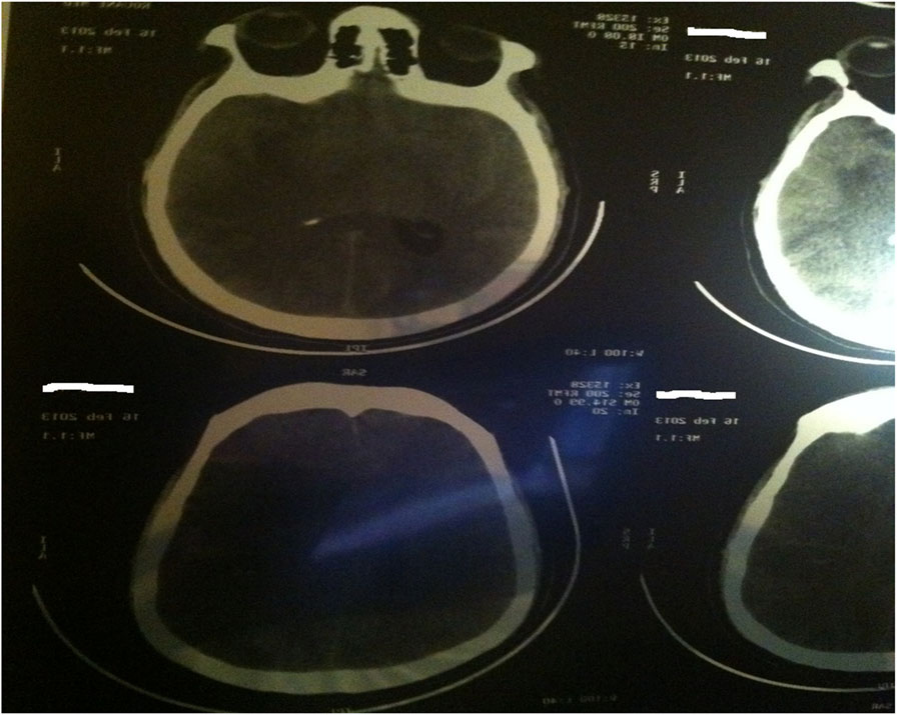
Cerebral computed tomography showing frontal, temporal, and parietal left ischemic stroke
